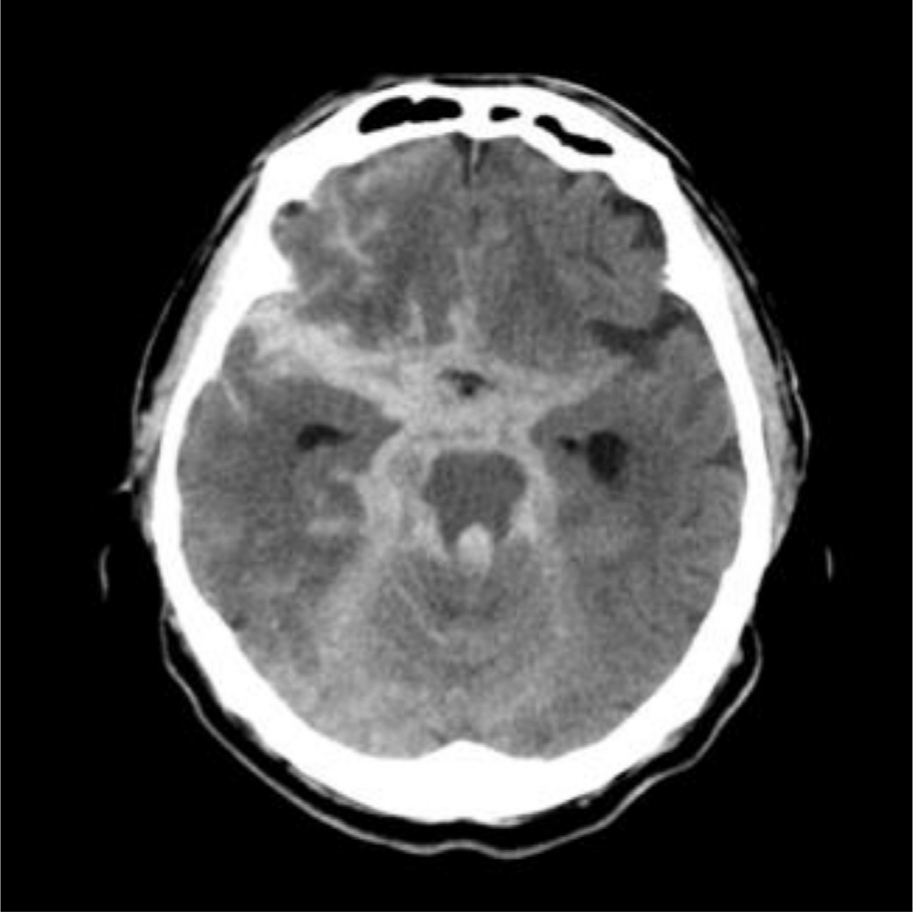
Computed tomography findings in an older patient with subarachnoid hemorrhage. The computed tomogram shows a thick subarachnoid hemorrhage, predominantly in the right ambient cistern.
